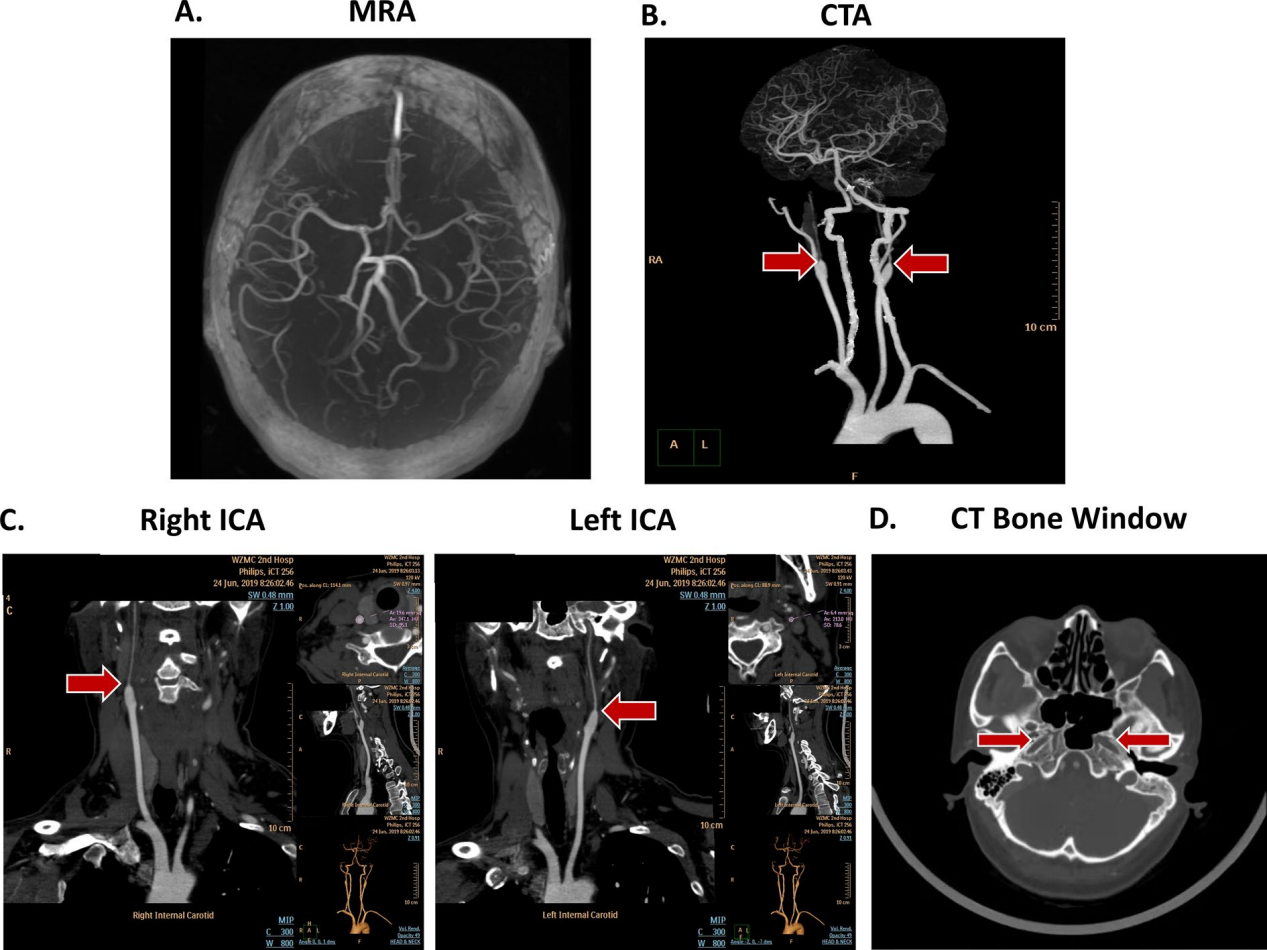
The neuroimaging examinations: A MRA; B CTA; C the re-established images of bilateral ICA. D CT bone window
